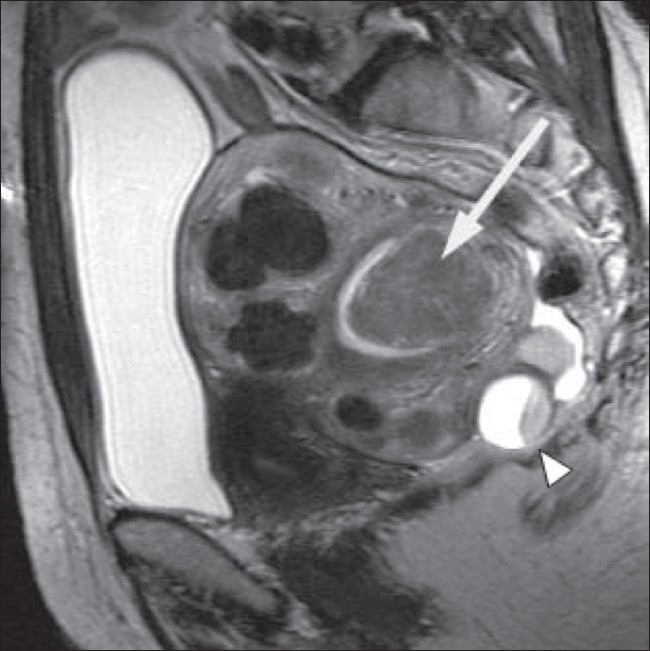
A 51-year-old woman with a history of menorrhagia. Sagittal T2W MRI shows a bulky retroverted uterus containing multiple intramural fibroids and a large submucosal fibroid (arrow) projecting into the endometrial cavity. A complex ovarian cyst is also incidentally demonstrated posterior to the uterus (arrowhead)
